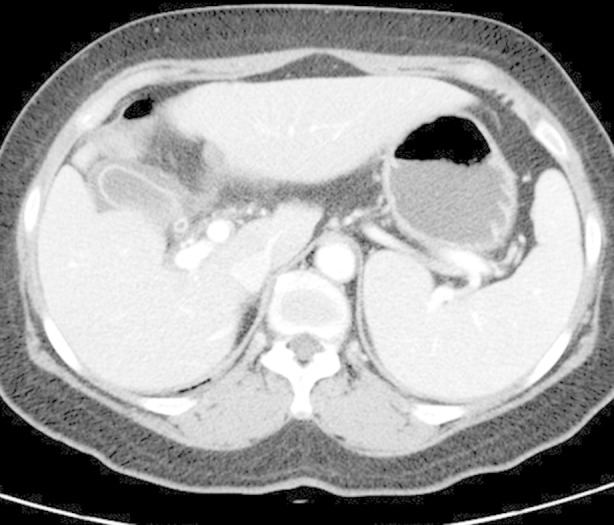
Scrub typhus in a 67-year-old woman. Serial contrast-enhanced abdominal CT images shows gallbladder wall thickening and splenomegaly.
